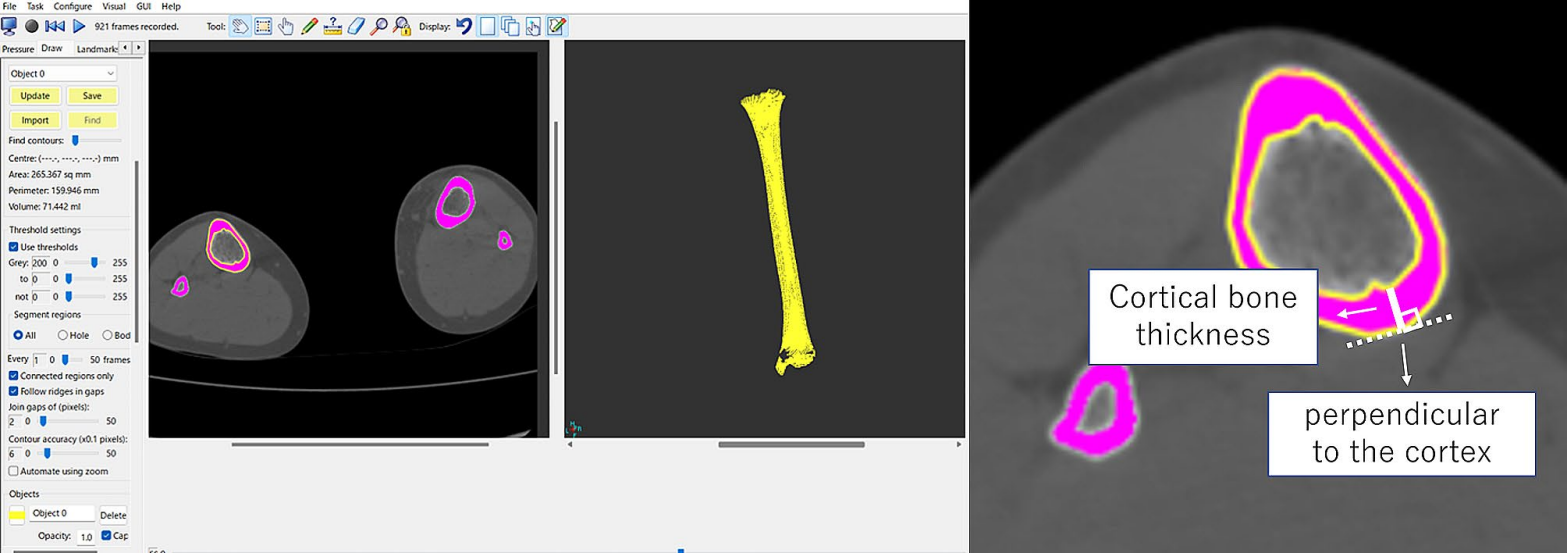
Automatic calculation in a 3D space using the high-resolution cortical thickness measurement from clinical CT data This technique relies on a mathematical model of the anatomy and imaging system that is fitted to data at a large number of sites around the tibia. Given the prior segmentation of the tibial diaphysis, CT values were examined along short lines that straddled and were perpendicular to the cortex
